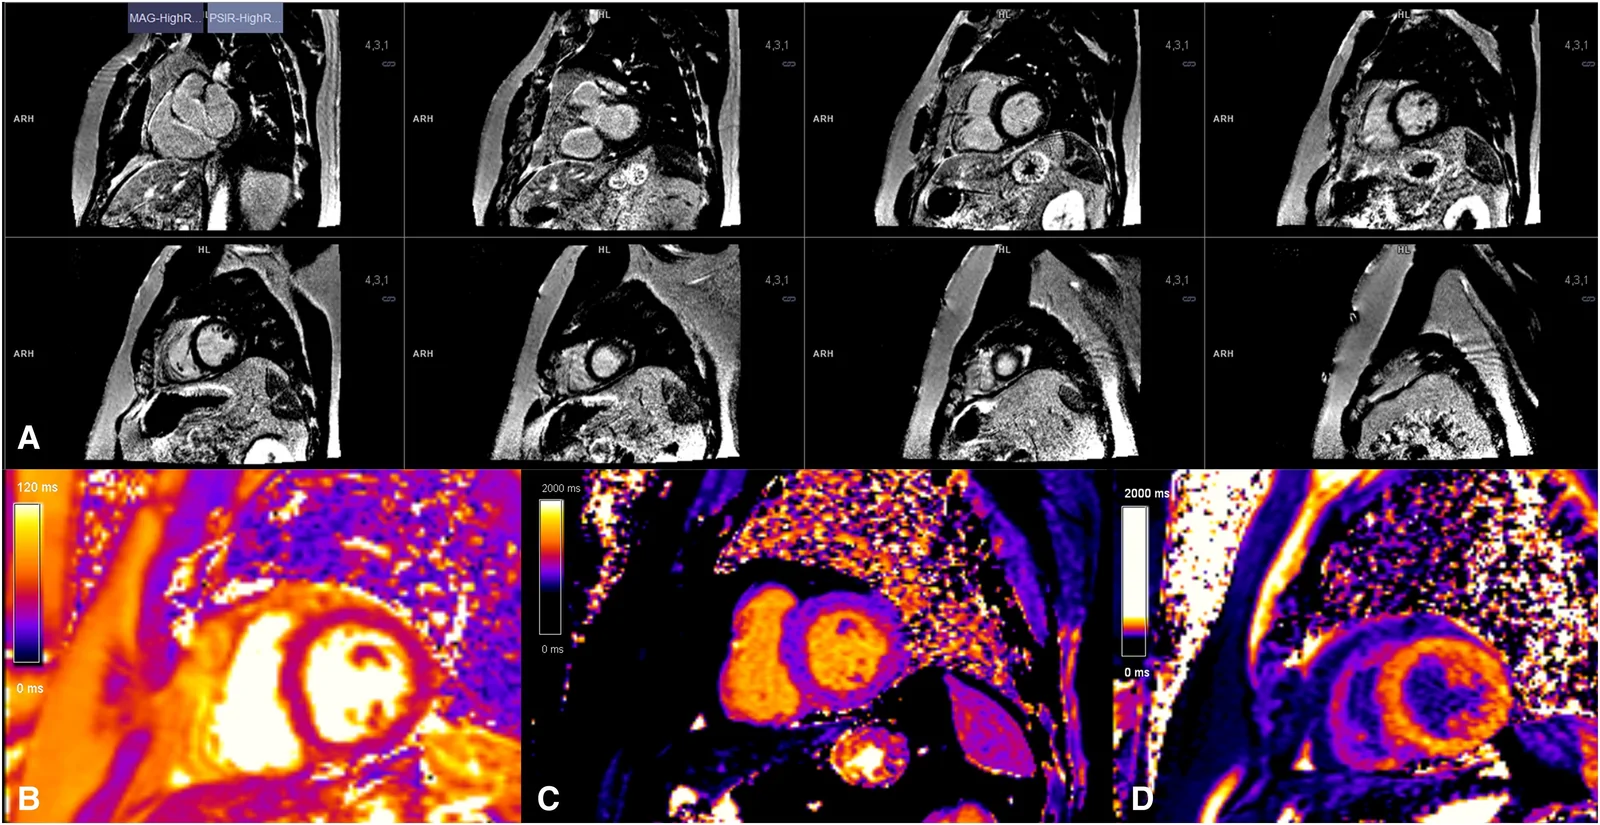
Cardiac magnetic resonance imaging demonstrating preserved myocardial tissue characteristics. (*A*) Late gadolinium enhancement imaging using a phase-sensitive inversion recovery sequence in short-axis view showing no evidence of myocardial fibrosis. (*B*) T2 myocardial mapping (short-axis, mid-ventricular level) without signs of myocardial oedema. (*C*) Native T1 myocardial mapping (short-axis, mid-ventricular level) demonstrating normal myocardial T1 values. (*D*) Post-contrast T1 myocardial mapping (short-axis, mid-ventricular level). Extracellular volume values were within the normal range, indicating the absence of diffuse myocardial fibrosis.
